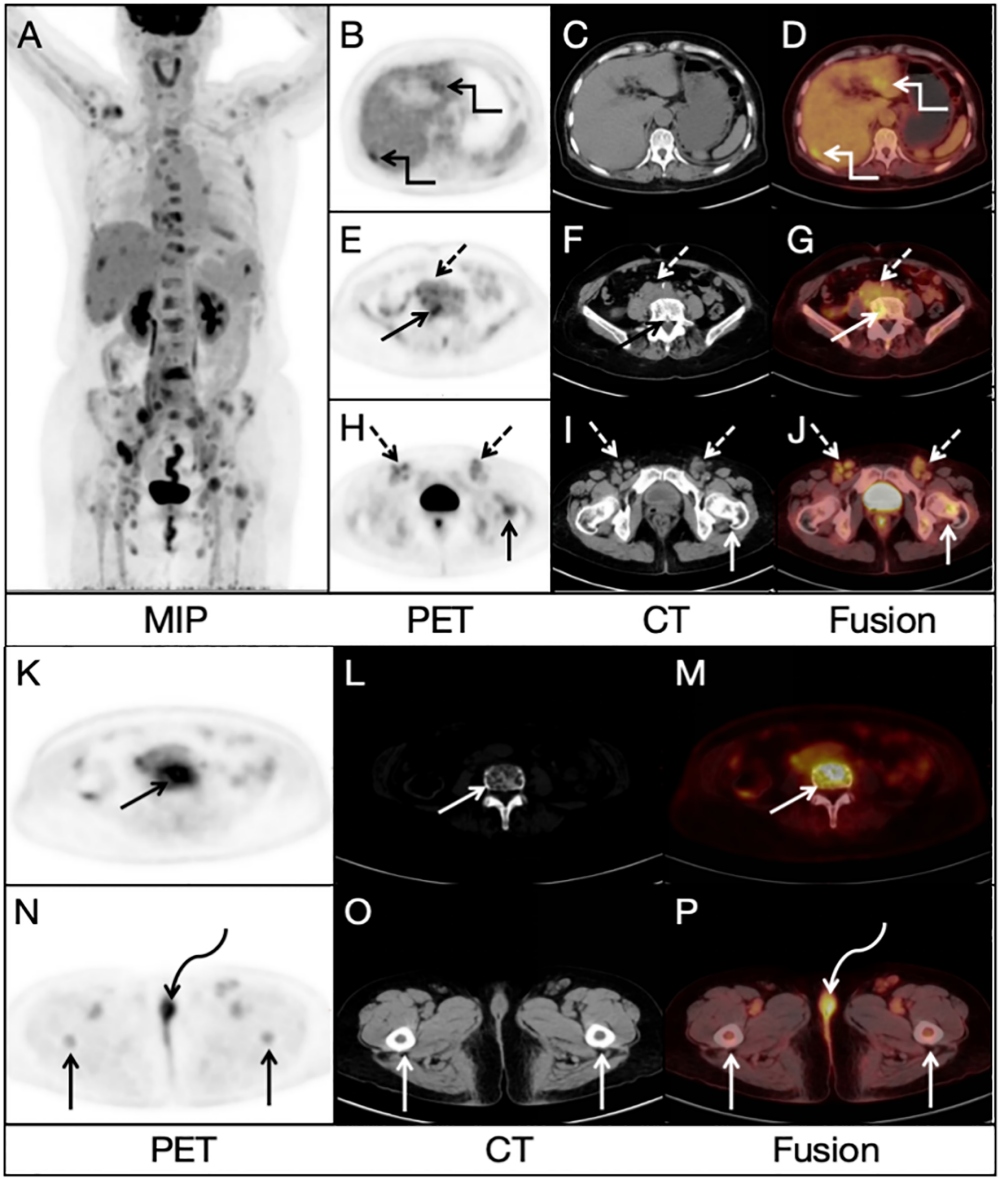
Radiological findings on ^18^FDG PET/CT image: (A) Multiple lesions in the bones, liver and lymph nodes. (B, D) Multiple foci of abnormal activity (broken arrows) in the liver. (C) No clear evidence of anatomical abnormality in the corresponding area on CT image. (E–J) Enlarged retroperitoneal and bilateral inguinal lymph nodes (dashed arrows). (K–M) Multiple hypermetabolic foci (solid arrows) in the bones (N, P) An additional focal activity (curved arrows) with a SUV_max_ of 6.2 in the vulva. (O) No clear evidence of anatomical abnormality in the corresponding area on CT image.
